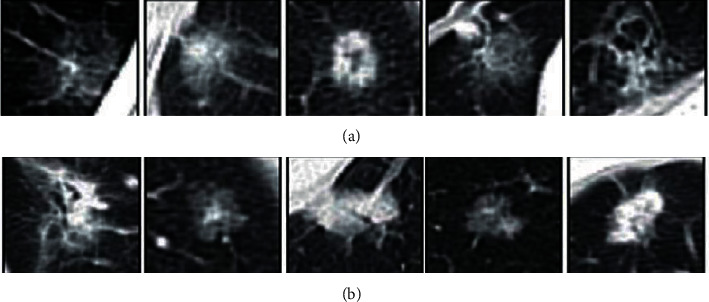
CT images with confirmed IAC and MIA [2]. (a) IAC. (b) MIA.
